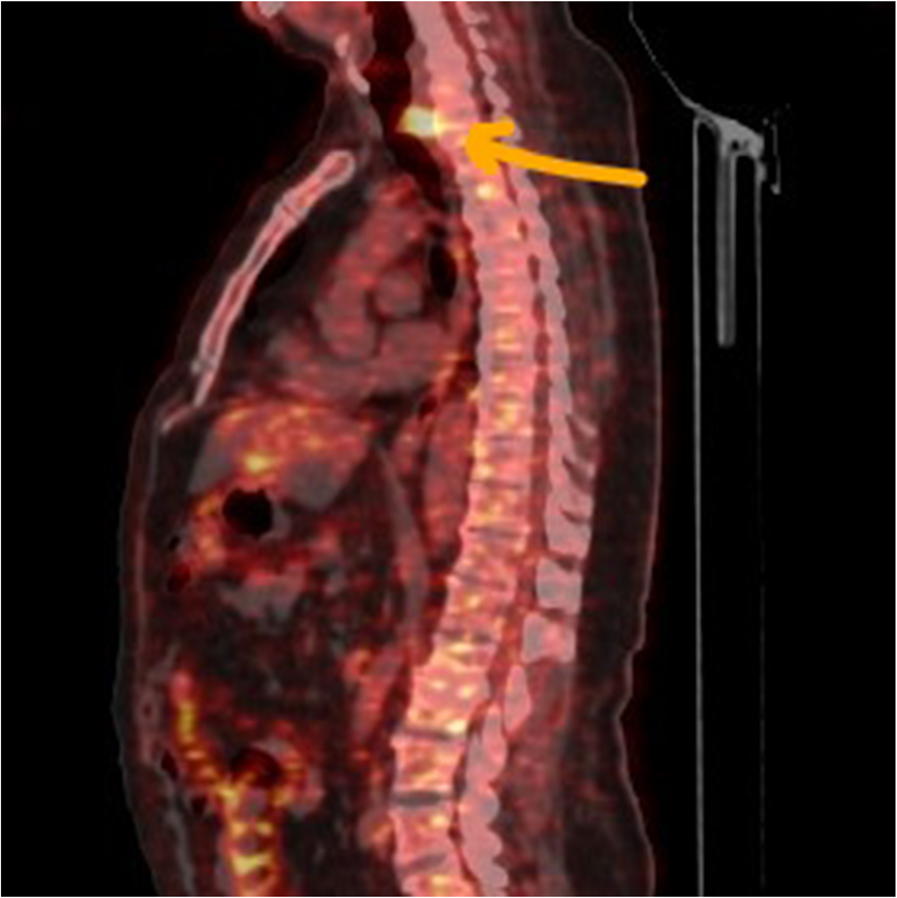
PET-CT sagittal view showing hyperactive lesion with an SUV of 6.5
